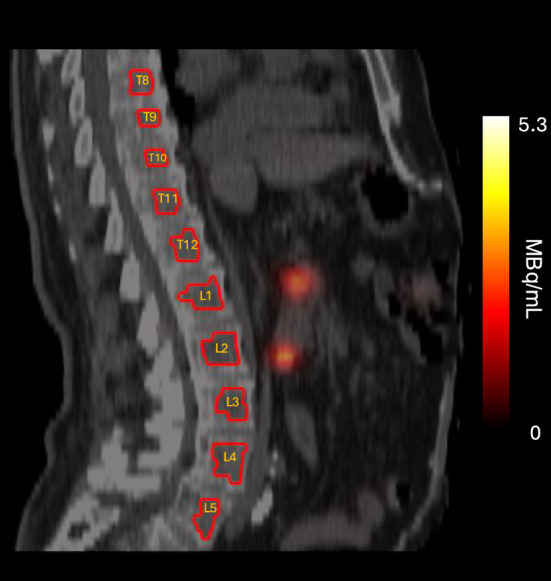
SPECT/CT image of patient K, with the thoracic and lumbar vertebrae VOI delineated in red.
